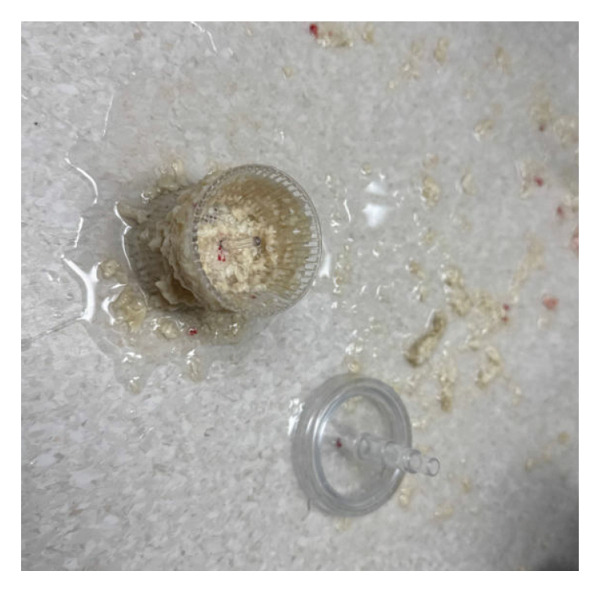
Aspirated candidal bezoar, showing the aspirated candidal bezoar obtained during a bilateral percutaneous nephroscopy with ultrasonic lithotripsy.
